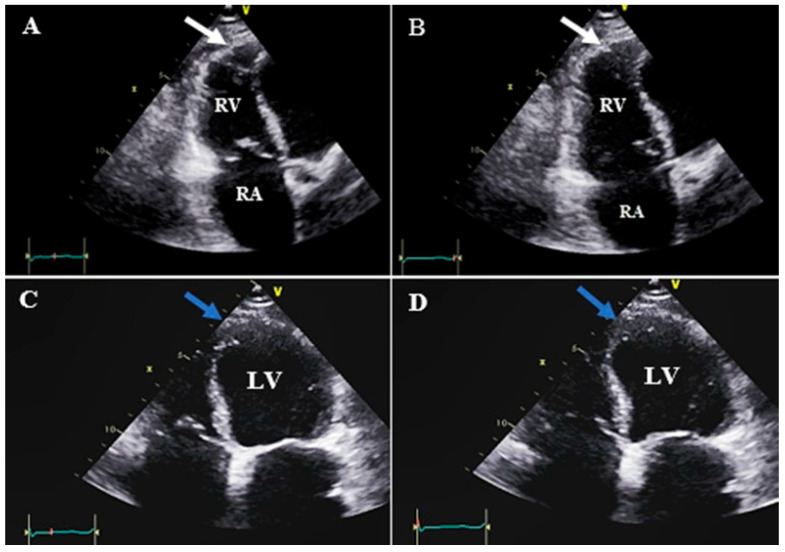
Right and left ventricular apical and apicolateral aneurysms in the apical RV focus view (white arrow) and apical four chamber view (blue arrow) with no clot (related to case #1); (A,C) end systole, (B,D) end diastole. Abbreviations: LV: left ventricle; RA: right atrium; RV: right ventricle.
